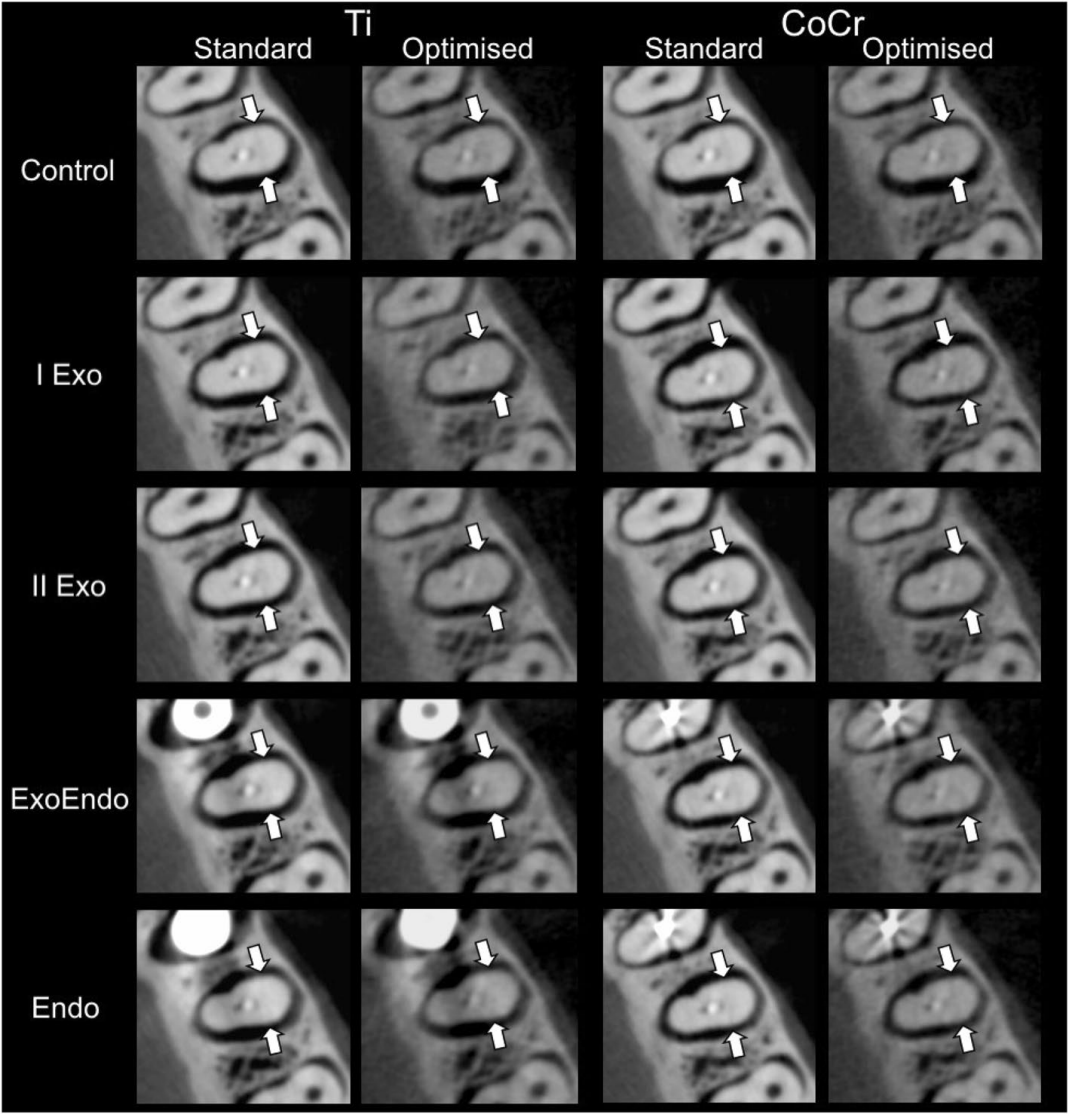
Cropped axial reconstructions of the standard and optimised CBCT protocols in different dispositions of titanium implants and cobalt-chromium intracanal posts in the mandible. The white arrows highlight the vertical root fracture in the second premolar.
